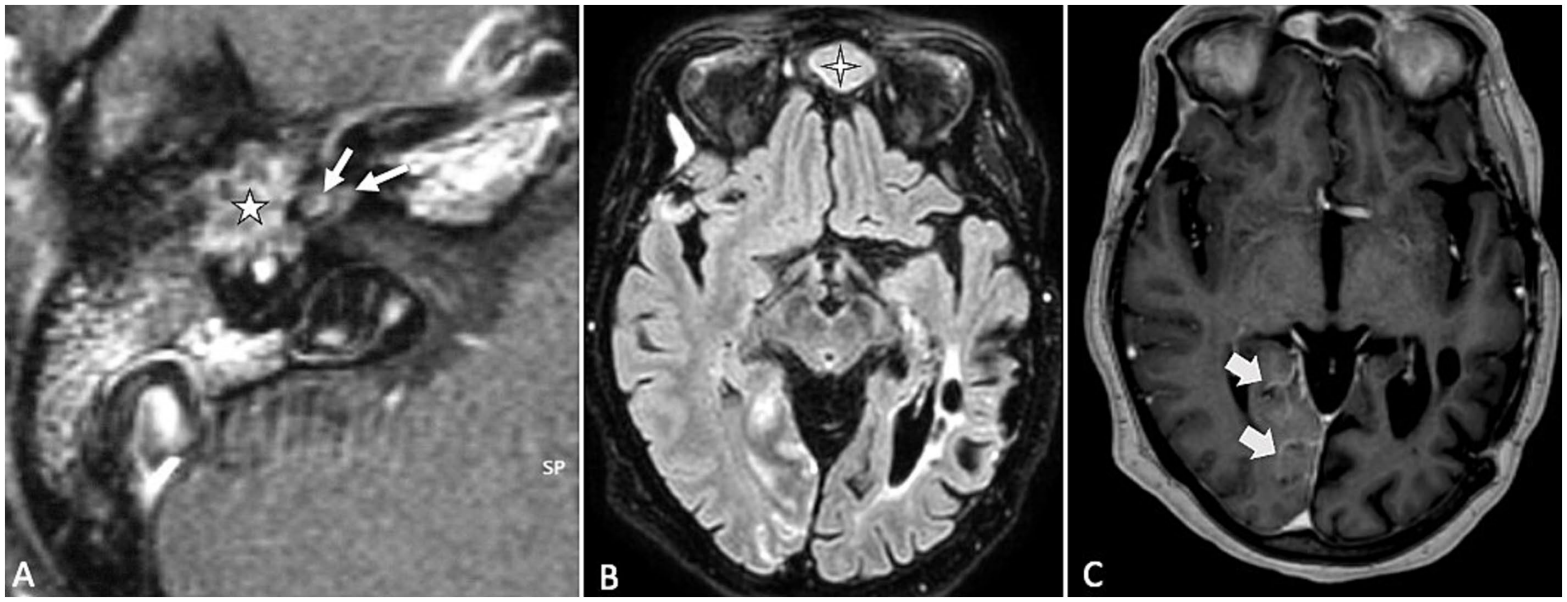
(A) Contrast-enhanced MRI, T1-weighted sequence showing acute otitis media with inflammatory enhancing granulation tissue filling of the right middle ear cavity (star) and suppurative labyrinthitis with non-nodular contrast enhancement (thin arrows) in the basal and second turns of the cochlea. (B,C) Another patient: FLAIR and T1-weighted sequences post-gadolinium injection reveal frontal sinusitis (cross symbol) and leptomeningeal enhancement (large arrow) in the right occipital region, consistent with occipital leptomeningitis.
